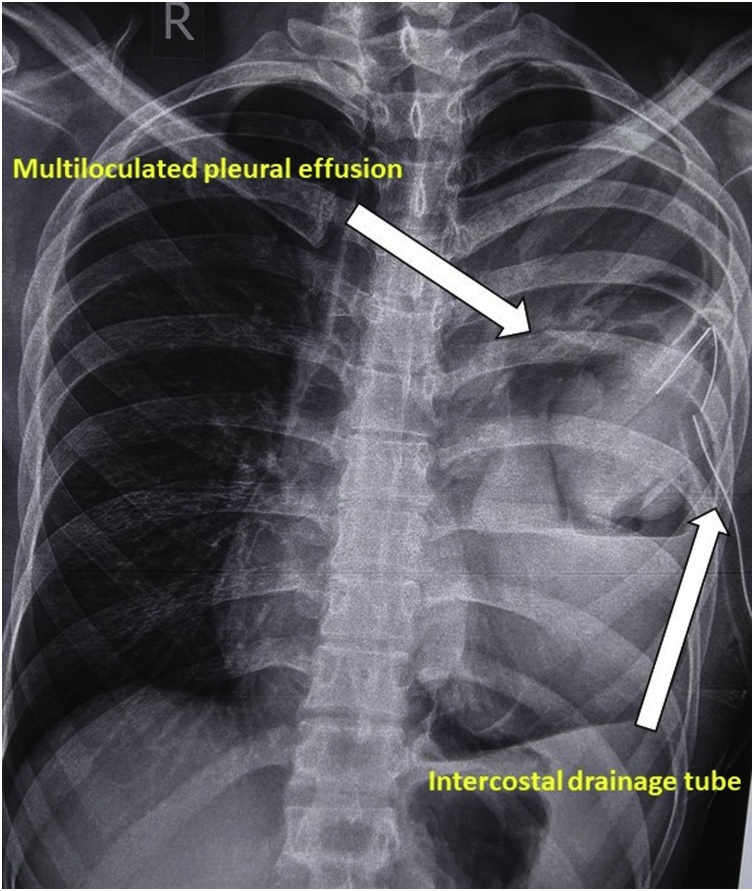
Chest radiograph showing multiloculated pleural effusion with intercostal drainage tube in situ.
